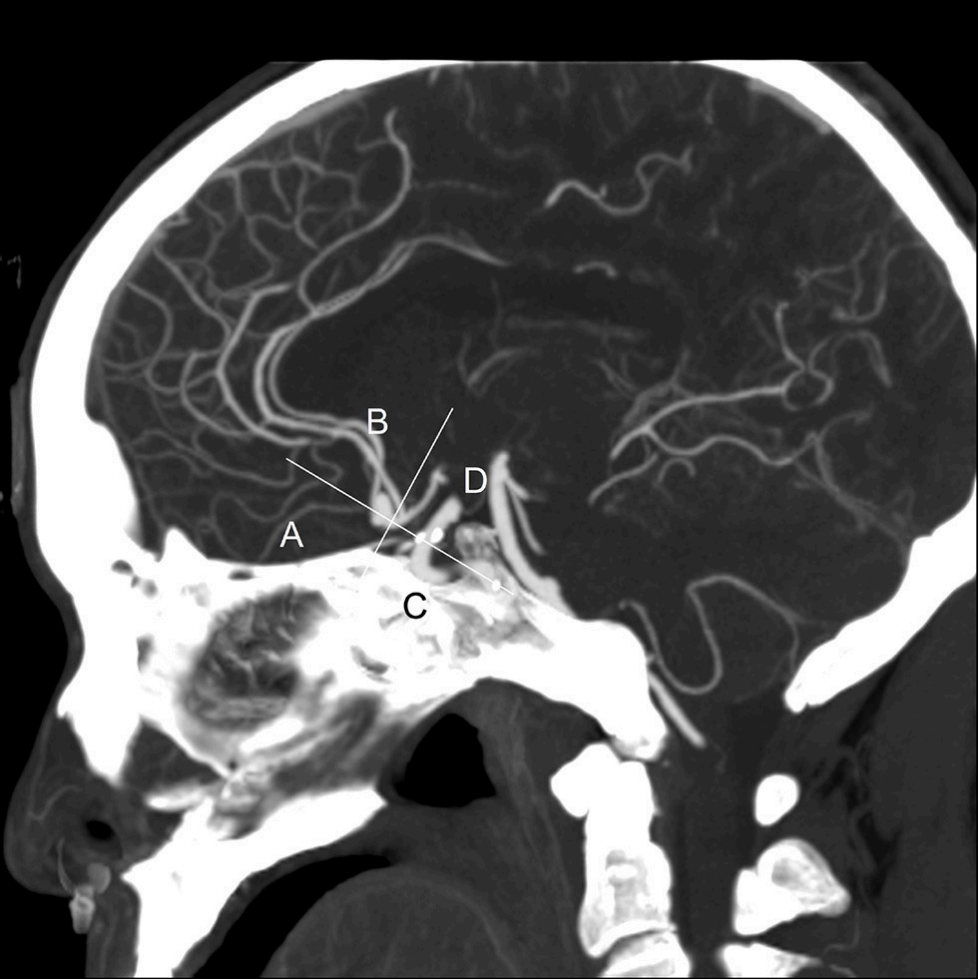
This is a sagittal maximum intensity projection (MIP) of a computed tomography angiography (CTA) from a typical patient. The directions of aneurysm dome were defined as anterior–inferior (A), anterior–superior (B), posterior–inferior (C), and posterior–superior (D). This picture depicts the direction of the aneurysm dome as B.
